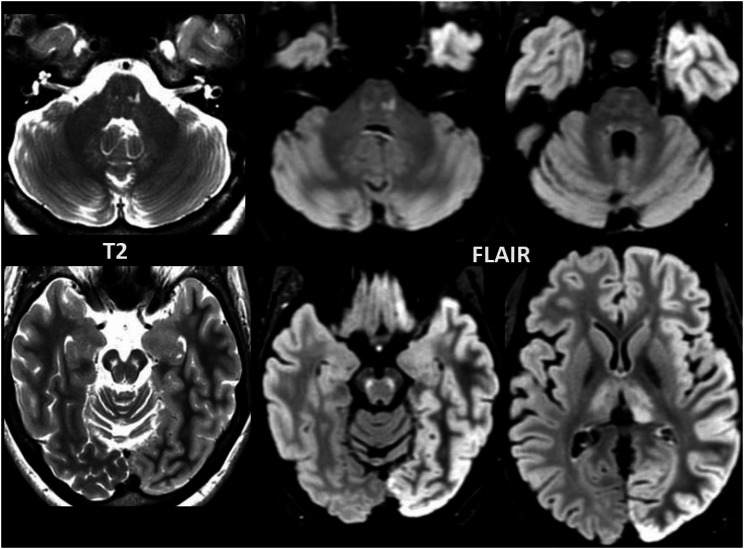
T2-weighted and FLAIR images of patient 8 are shown here. She presented with a (transient) stroke-like episode of aphasia, apraxia and hemianopsia, reflected by the cortical hyperintensity and swelling of the left cerebral hemisphere and reactive hyperintensity of the left pulvinar. The abnormalities in the pons are subtle and punctate in this patient, additionally the signal of the dentate nucleus is abnormal. The red nucleus and thalamus are involved
